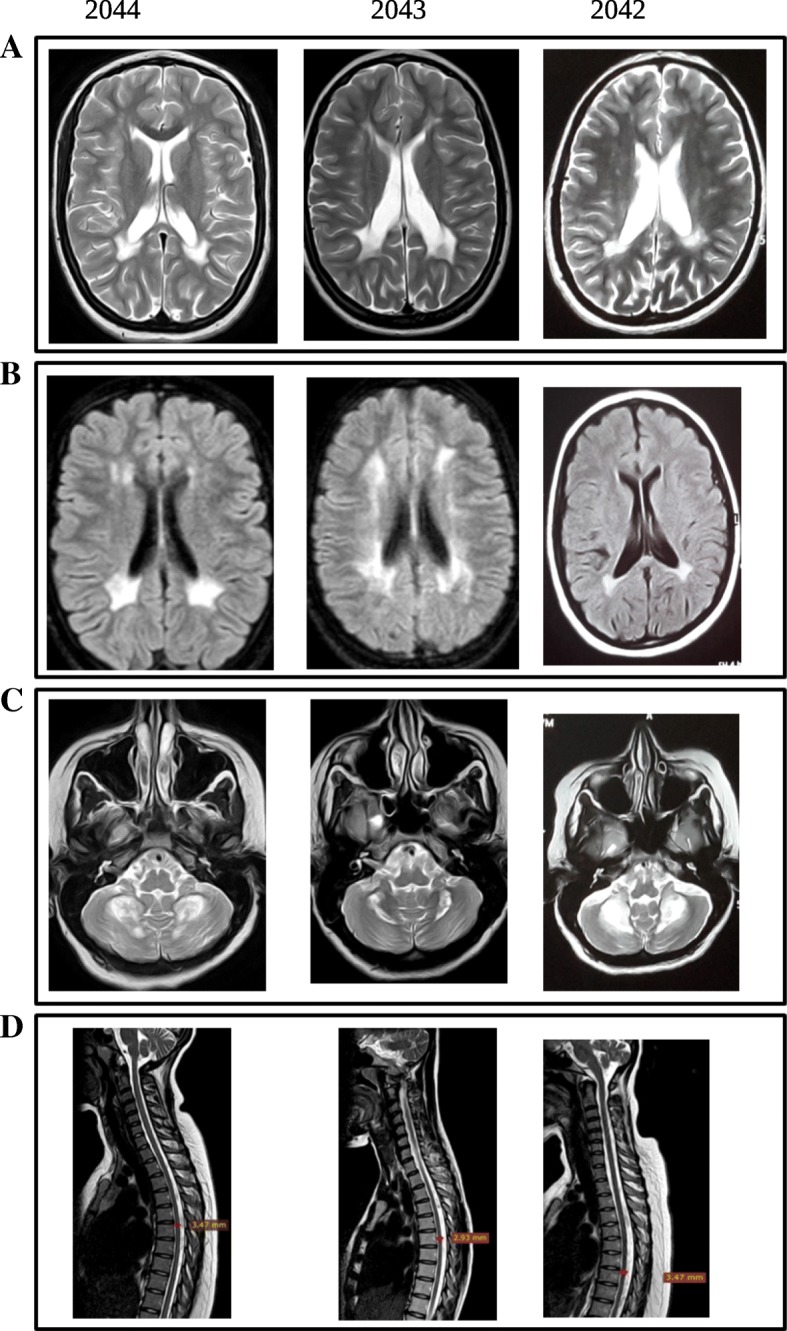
Brain and spinal cord MRIs of the three patients. MRIs of subjects 2044, 2043 and 2042 ordered from left to right. a and b Axial T2 and fluid-attenuated inversion recovery (FLAIR) sections of brain MRIs showing abnormal periventricular white matter high signal intensities in the three subjects. c Axial T2 MRIs through the cerebellar hemispheres demonstrate mild cerebellar atrophy in the three subjects. d Sagittal T2 MRIs of the spinal cord show spinal cord atrophic changes in the three subjects
